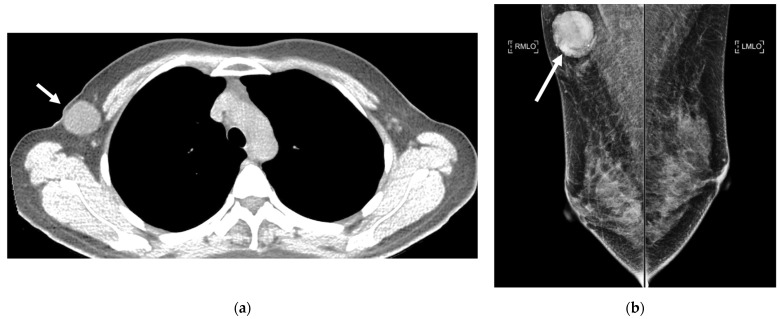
An incidental breast mass in a low-dose computed tomograph (LDCT). (a) An axial LDCT of a 48-year-old female demonstrates a 3.3 cm right breast mass (arrow). (b) A mammogram revealing a corresponding mass at the right upper breast (arrow), which was subsequently confirmed as a benign phyllodes tumor by surgery and pathology. (RMLO, right mediolateral oblique view. LMLO, left mediolateral oblique view).
